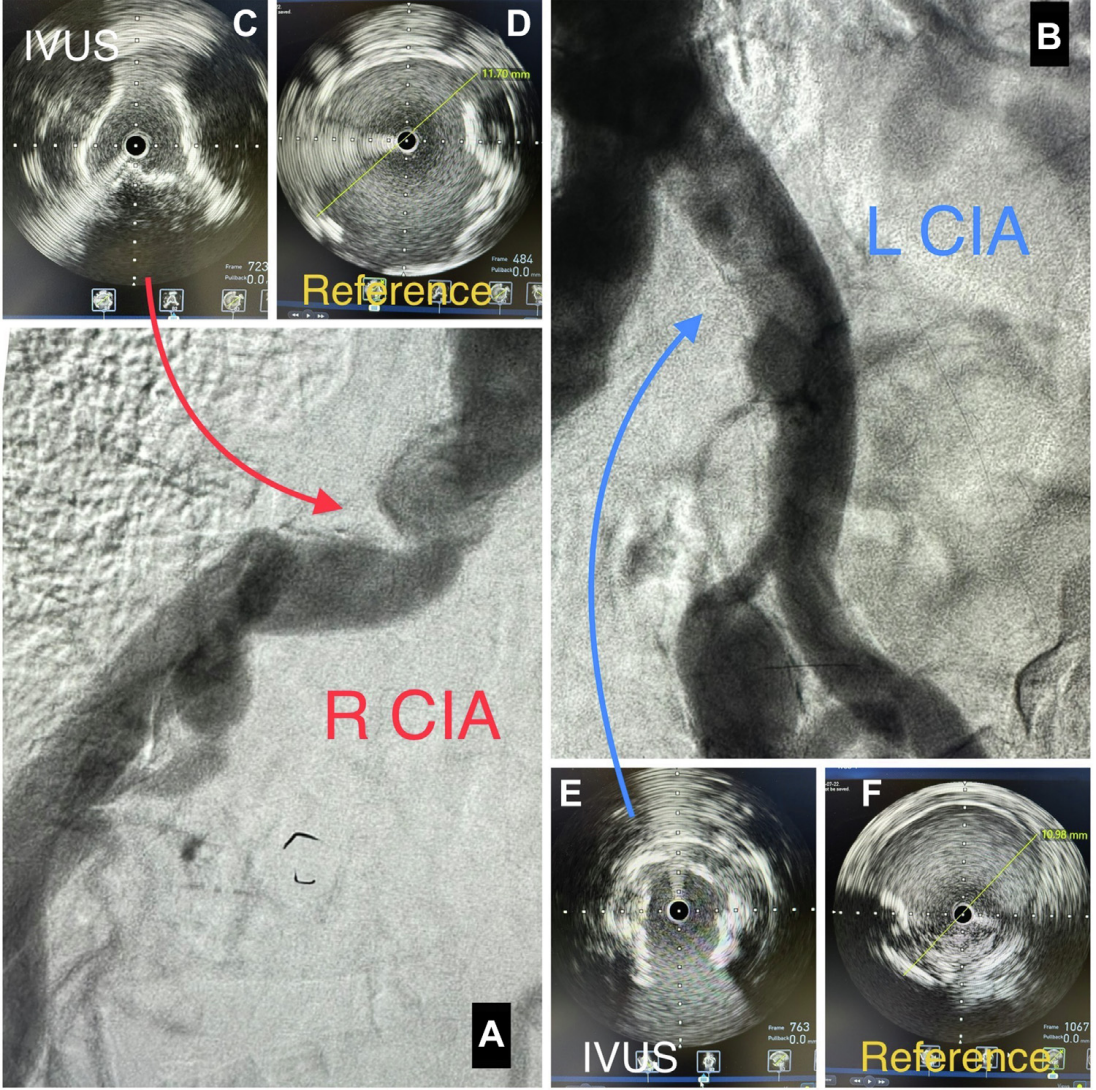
Diagnostic imaging of bilateral iliac arteries. (A) Diagnostic angiogram of the right common iliac artery (CIA). (B) Diagnostic angiogram of left CIA. (C) Intravascular ultrasound (IVUS) of severe, heavily calcified stenosis in the right CIA. (D) IVUS of the right CIA reference vessel. (E) IVUS of severe, heavily calcified stenosis in the left CIA. (F) IVUS of the left CIA reference vessel.
